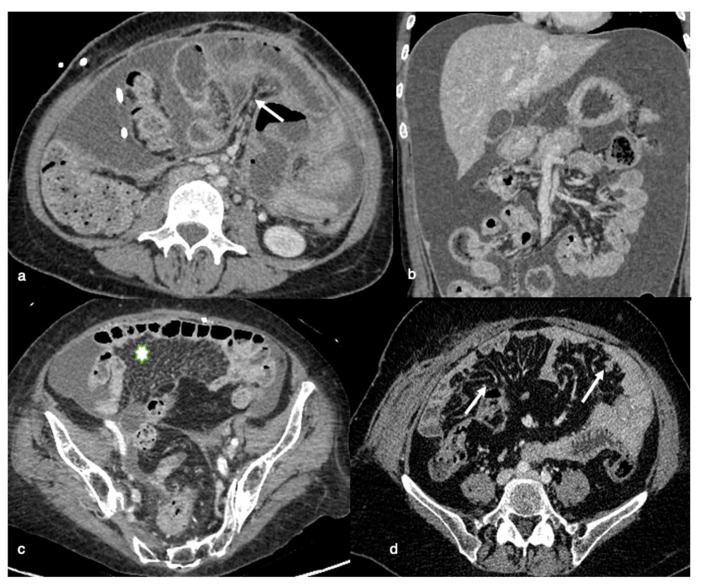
Infiltration of the mesentery. Axial contrast-enhanced image (a) shows a diffuse infiltration and thickening of mesenteric reflections (arrow) causing diffuse rigidity and retraction of the mesentery, with contraction of bowel toward the retroperitoneum causing a clumped appearance on the coronal CT image (b). Axial contrast-enhanced image shows the “stellate pattern” produced when straightened blood vessels held rigid by the thickened mesentery look like stars in the sky (asterisk in (c)); infiltration of the mesentery can also be represented by nodular visceral peritoneal thickening with multiple small nodules in the mesenteric layers (arrows in (d)).
